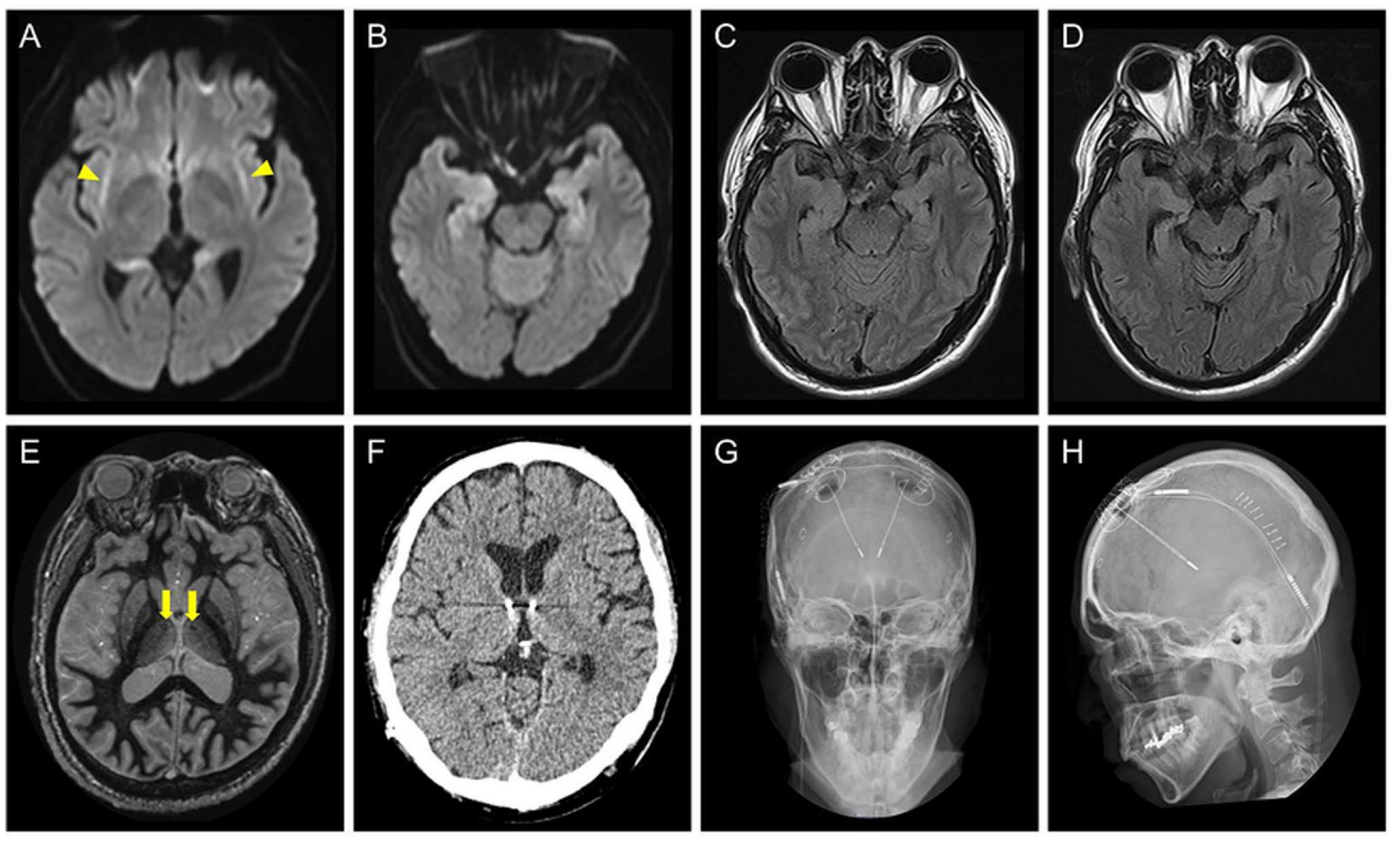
(A–C) Brain axial MRI of the patient at the age of 45 years, during the acute phase of cryptogenic new-onset refractory status epilepticus. Diffusion-weighted images show bilateral claustrum signs (A, yellow arrowheads) and bilateral hippocampal abnormalities (B). A fluid attenuated inversion recovery (FLAIR) image shows high signal intensity areas in the hippocampi (C). (D) The axial FLAIR image at the age of 51 years showing progression of atrophy of the bilateral hippocampi. (E) A preoperative fast gray matter acquisition T1 inversion recovery sequence at the age of 52 years shows the intact mammillothalamic tract (yellow arrows). (F–H) Postoperative brain axial CT (F) and skull radiographic (G,H) images showing placement of the stimulation leads.
